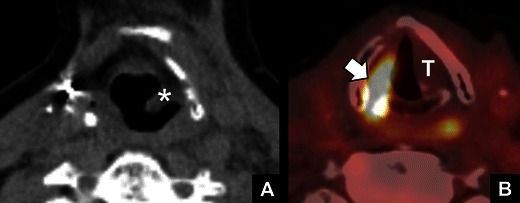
FDG-PET findings in VCP.*
